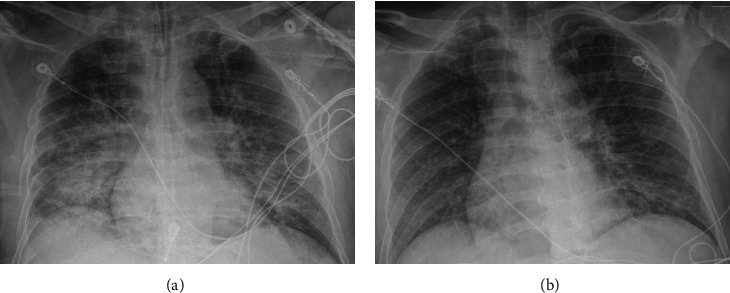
Chest X-rays. Chest X-ray (CXR) which was taken 2.5 hours after intubation (day 2) showed significant diffuse bilateral opacities. (a) CXR next day after extubation (day 7) showed improved bilateral opacities. (b)
